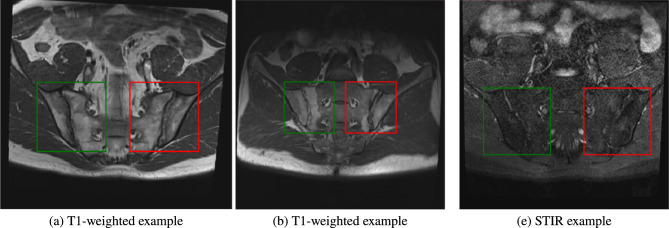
Example ROIs in different scans in the dataset with their marked-up annotated landmarks. (a, b) T1-weighted examples while (c) is a STIR example. Depending on the FOV of the scan, the SIJs can vary in size which is why ROI detection is crucial in our case. For example, the SIJ in (b) is relatively smaller when compared to the SIJs in (a, c). The right SIJs are shown in green bounding boxes while the left SIJs are shown in red bounding boxes. Although we show a single slice here, the ROIs cover the 3D volume of a given scan sequence (T1-weighted or STIR) but remain static in spatial coordinates.
